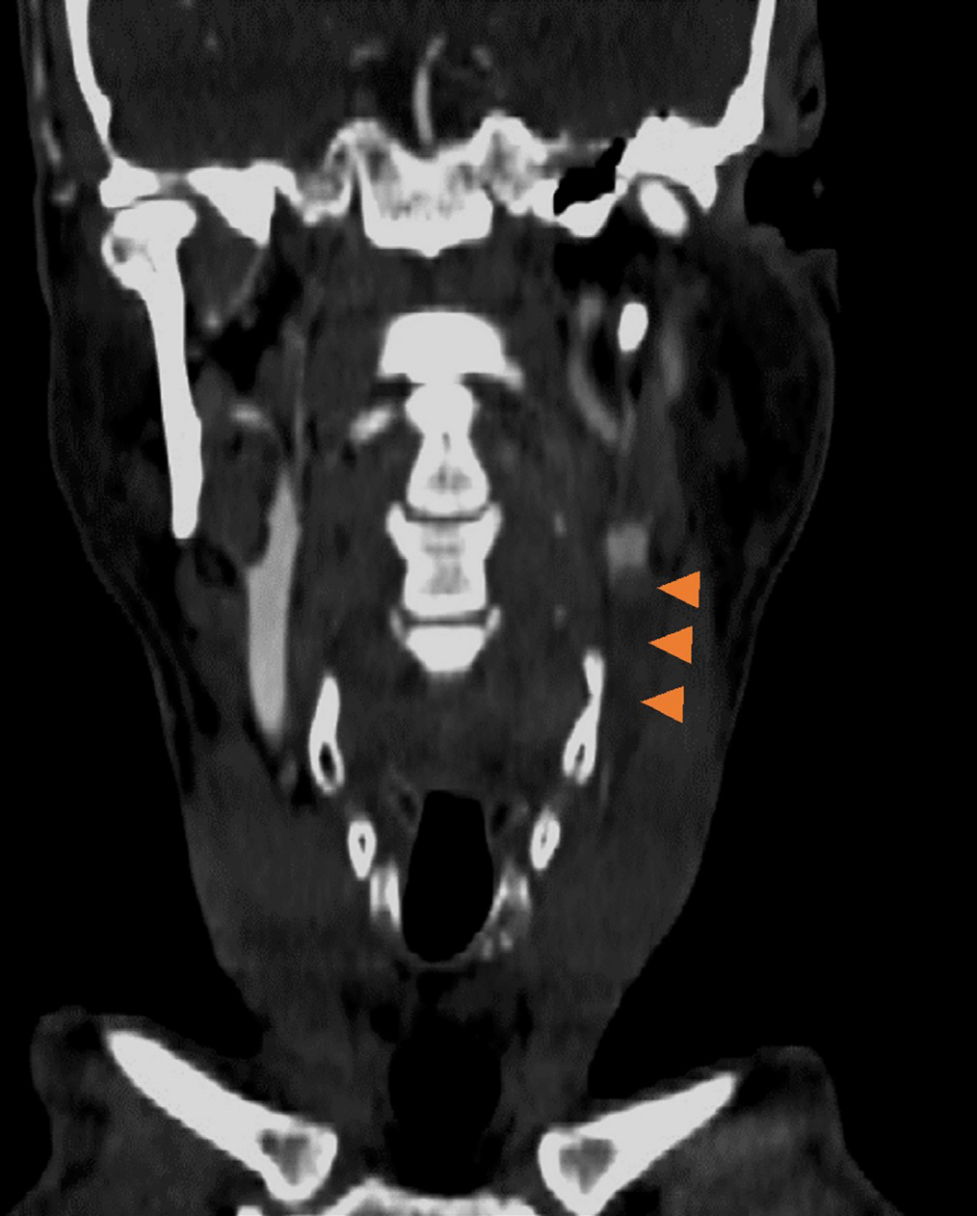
Coronal CT carotid angiogram shows absent filling of the left common carotid artery.
